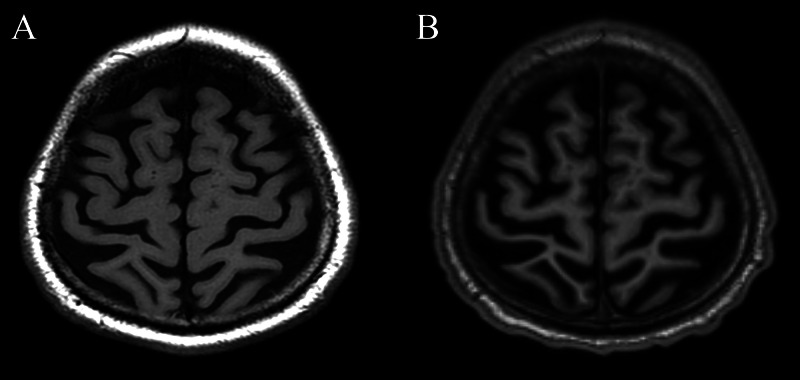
(A) Axial T1-weighted images from the initial MRI and (B) repeat MRI performed one year after initial MRI shows interval progression of peri-Rolandic atrophy (arrows), greater in the right hemisphere.
